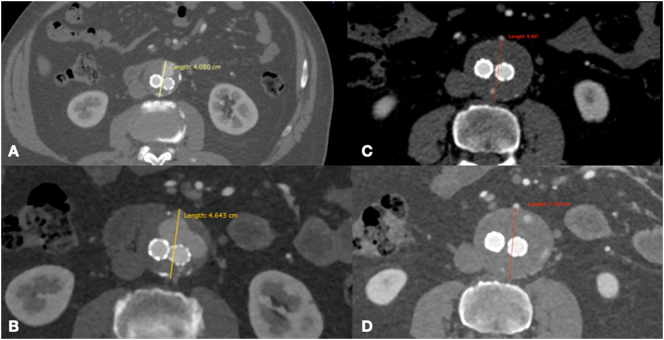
CT-angiography at 6 months showing a worsening of type 2 endoleak (A and B) with growth of AAA greater of 0.5 cm (C and D).
